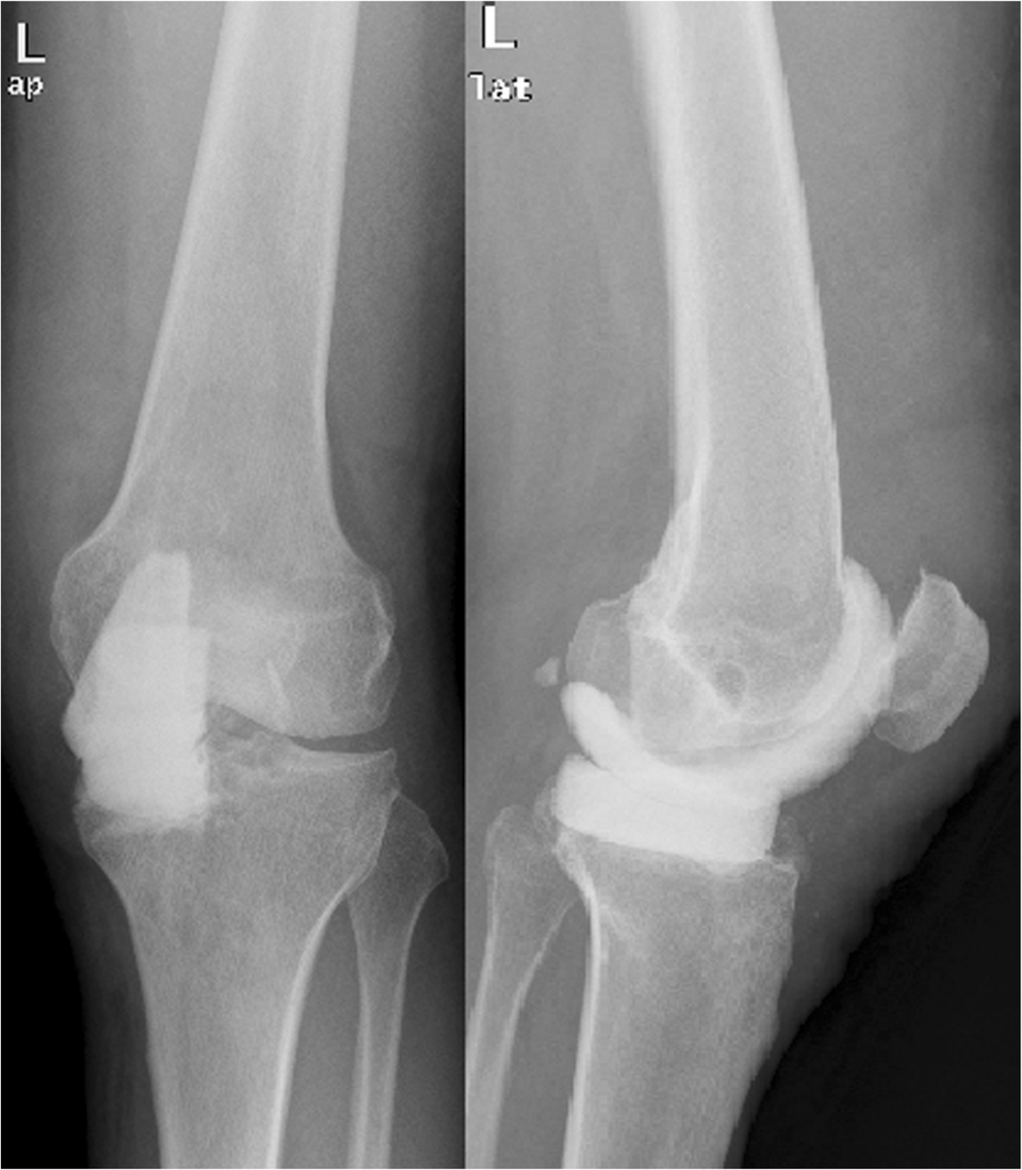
X-rays (AP and lateral view) after 1^st^ stage of two-stage revision arthroplasty (Spacer implantation)
